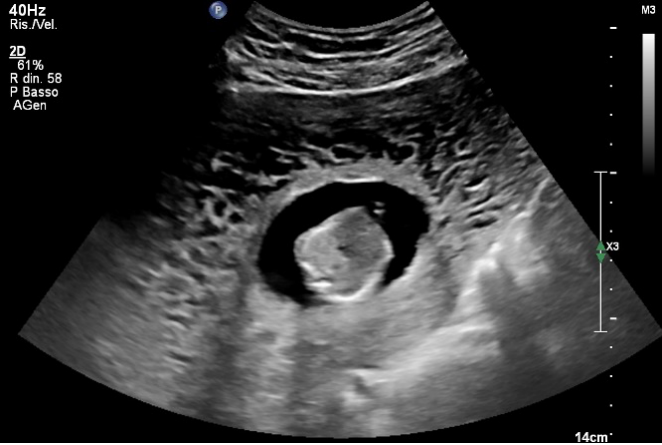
Ultrasound scan at 19 weeks of gestation showing diffusely thickened myometrium with anechogenic areas of larger size (Swiss-cheese appearance).
